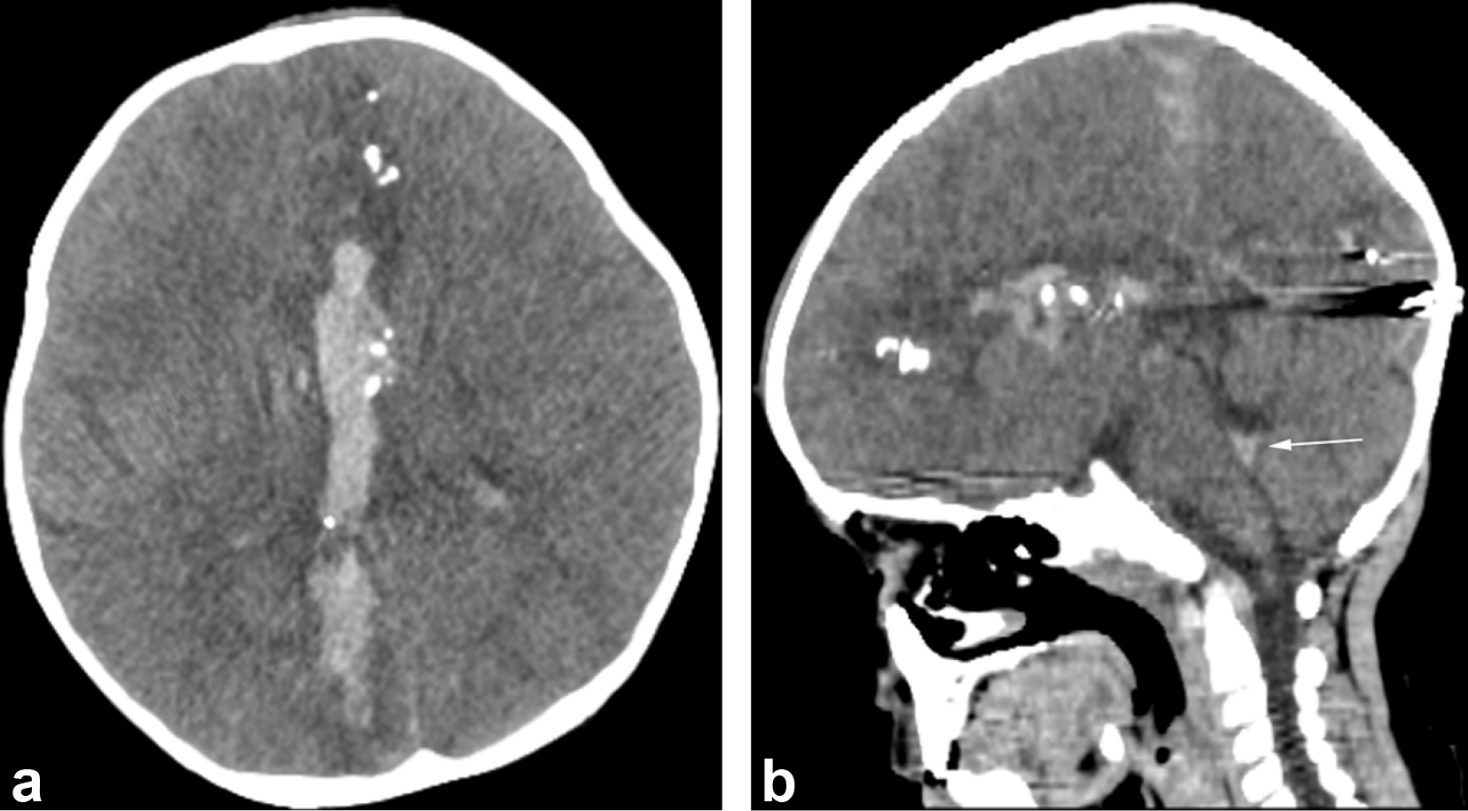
Initial non-contrast CT scan of the head. (a) The axial section shows an intracranial hemorrhage from the left frontal to right occipital lobe with bone and metal fragments found along the path, and metal-density (HU 4000) at the right occipital region. (b) The sagittal section shows that the projectile path occurred at the level of the lateral ventricle, with a foreign body at the occipital region. Note that there is blood in the fourth ventricle as well (arrow). HU, Hounsfield unit.
